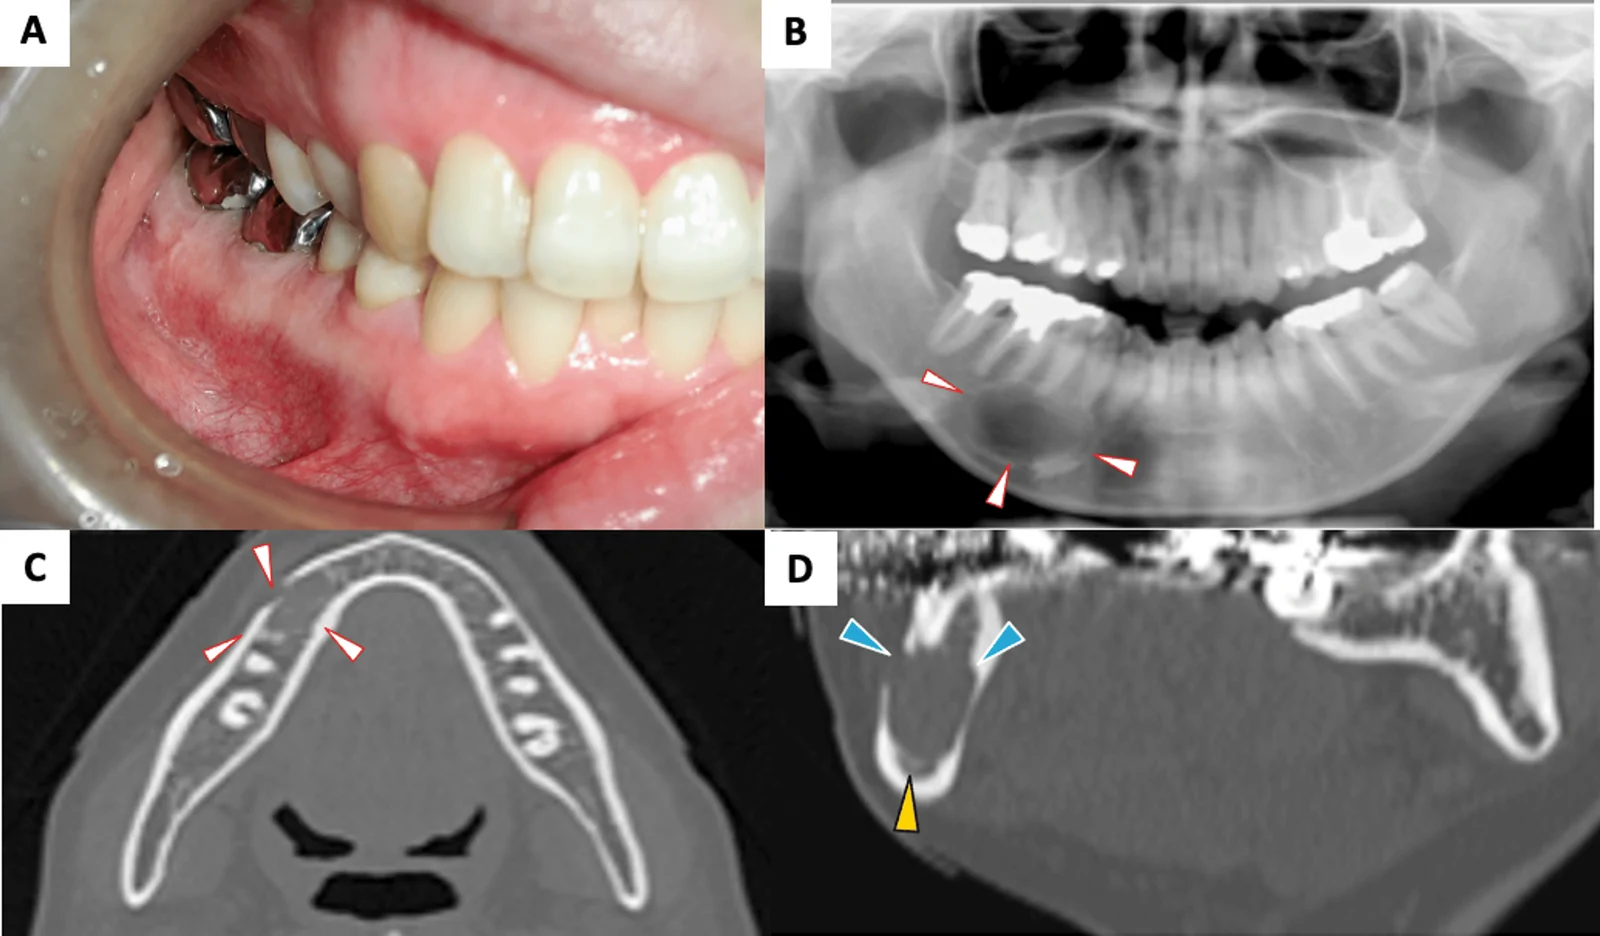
Clinical and radiographic findings at the initial visit. (A) Intraoral photograph showing no obvious swelling, mucosal discoloration, or ulceration in the right mandibular premolar-to-molar region. (B) Panoramic radiograph showing a well-defined unilocular radiolucency extending from the right mandibular premolar region to the mesial root of the first molar (red arrowheads), without obvious root resorption. (C) Axial computed tomography image showing smooth thinning and resorption of the buccal and lingual cortices (red arrowheads). (D) Reformatted computed tomography image showing enlargement of the mental foramen (blue arrowheads) and inferior displacement of the mandibular canal (yellow arrowhead).
